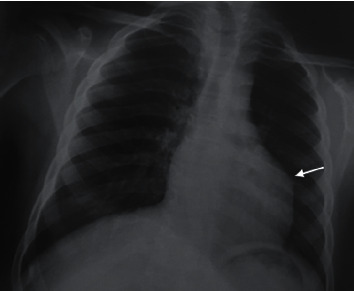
Frontal chest X-ray: globular appearance of the lower left arch (arrow).
